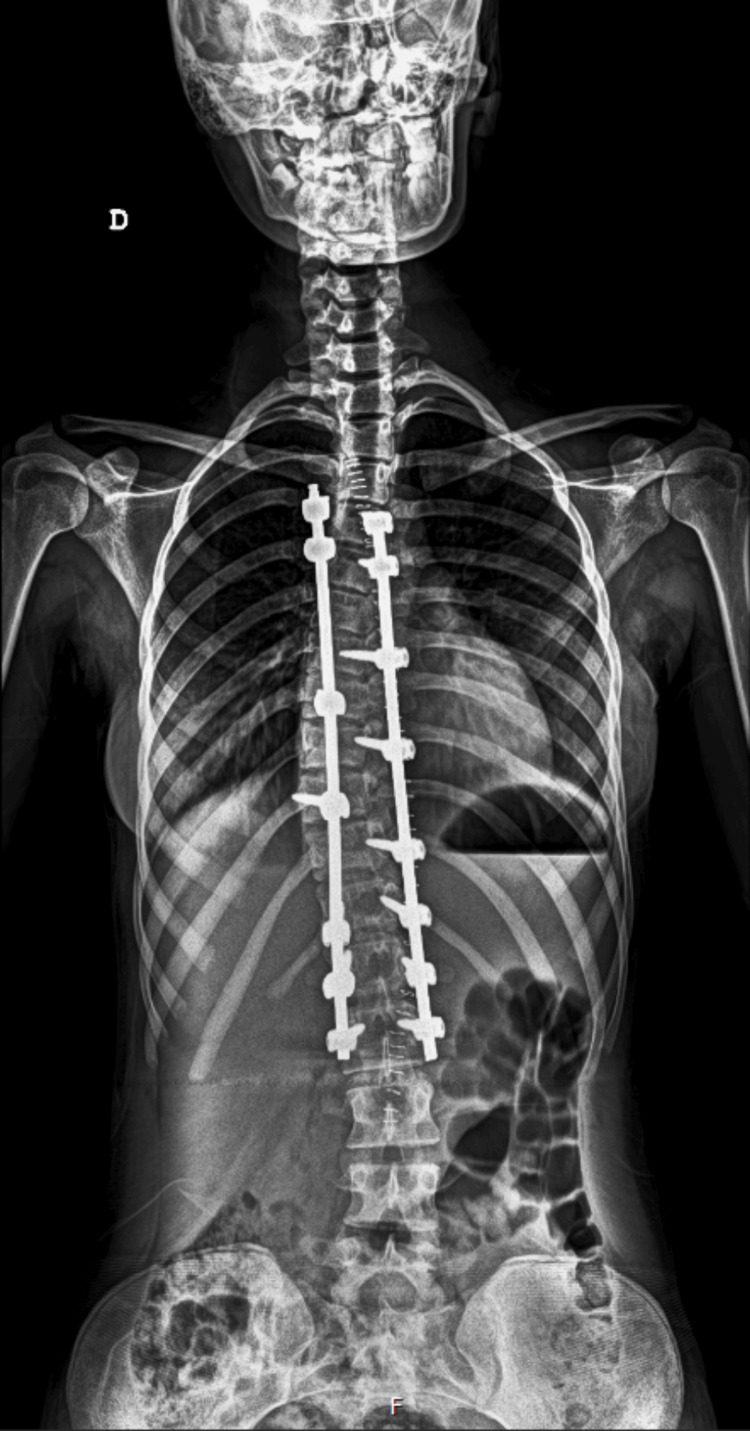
The patient's X-ray at discharge
